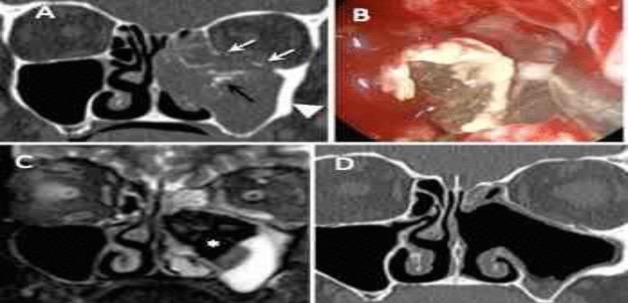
Coronal computed tomographic scans of the paranasal sinuses; A) a heterogeneous opacity of the left maxillary sinus, nasal cavity, and ethmoid air cells with microcalcification (black arrow) and sclerosis of the maxillary sinus wall (white arrowhead); phlogistic erosion of lamina papyracea and orbital floor is also seen (white arrows). Endoscopic removal of a fungus ball in the left maxillary sinus, B) Magnetic resonance imaging shows a signal void on coronal T2-weighted images (small star), C) hyperintense inflamed mucosa at the alveolar recess of the maxillary sinus, D) coronal computed tomography image after surgery showing the complete resolution of fungus ball
